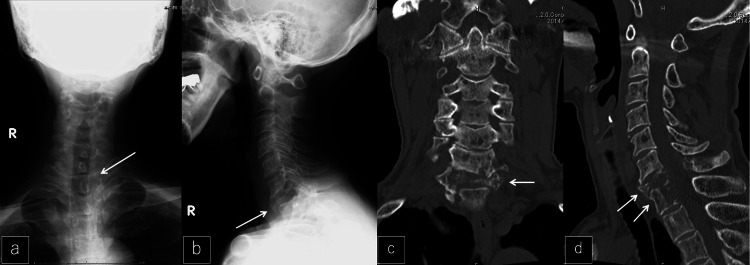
X-ray and CT imaging at the first visit a, b: Preoperative anteroposterior and lateral X-ray. The C7 vertebra was collapsed (white arrows). c, d: Coronal and sagittal CT. Decalcified bone in the posterior wall of C7 and T1 vertebrae (white arrows).
